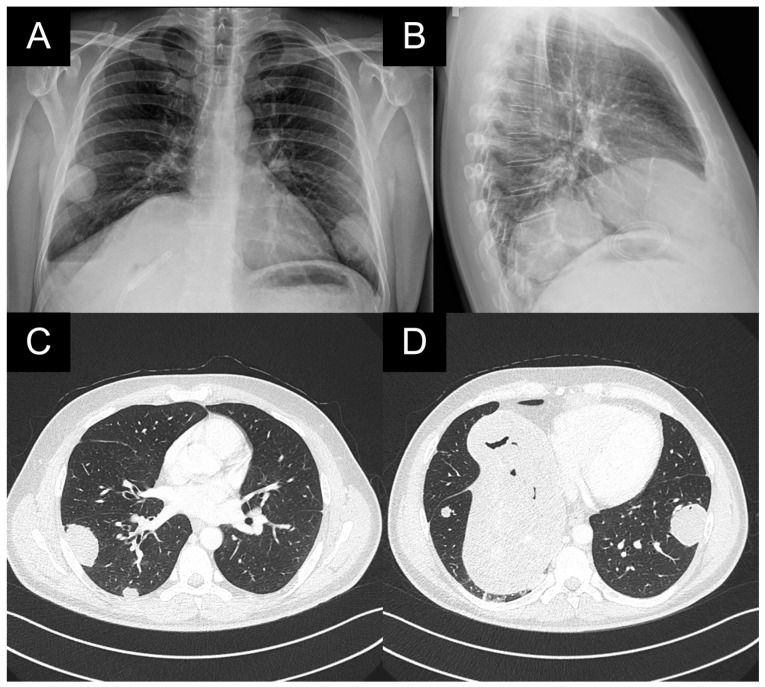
(A,B): Preoperative X-ray images of bilateral lesions in the lower lobes; (C): CT scan with the lesions in the right segments 6 and 8 measuring 4.6 and 1.4 cm; (D): 1 cm lesion in the 8th right segment and 4 cm lesion in the 9th left segment. An enlarged hepatic cyst percutaneously drained by hepatocentesis.
